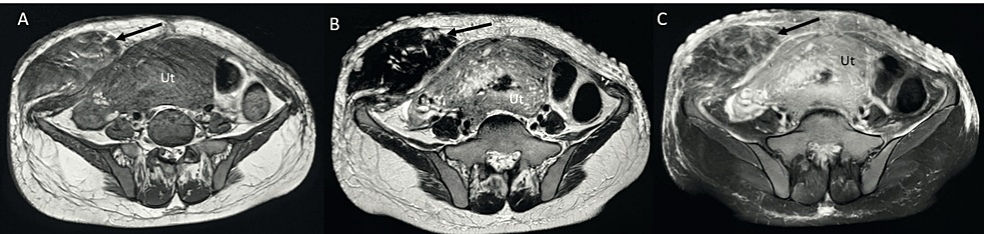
MRI T1W (A), T2 W (B), and fat-saturated T2W (c) images showing a large hematoma (arrows) involving the right side of the anterior abdominal wall involving subcutaneous tissue, right rectus abdominis, and right transversalis muscle. The lesion is isointense to the muscles on T1 and hypointense on T2-weighted images, suggesting acute hematoma. Note the bulky uterus (Ut).
